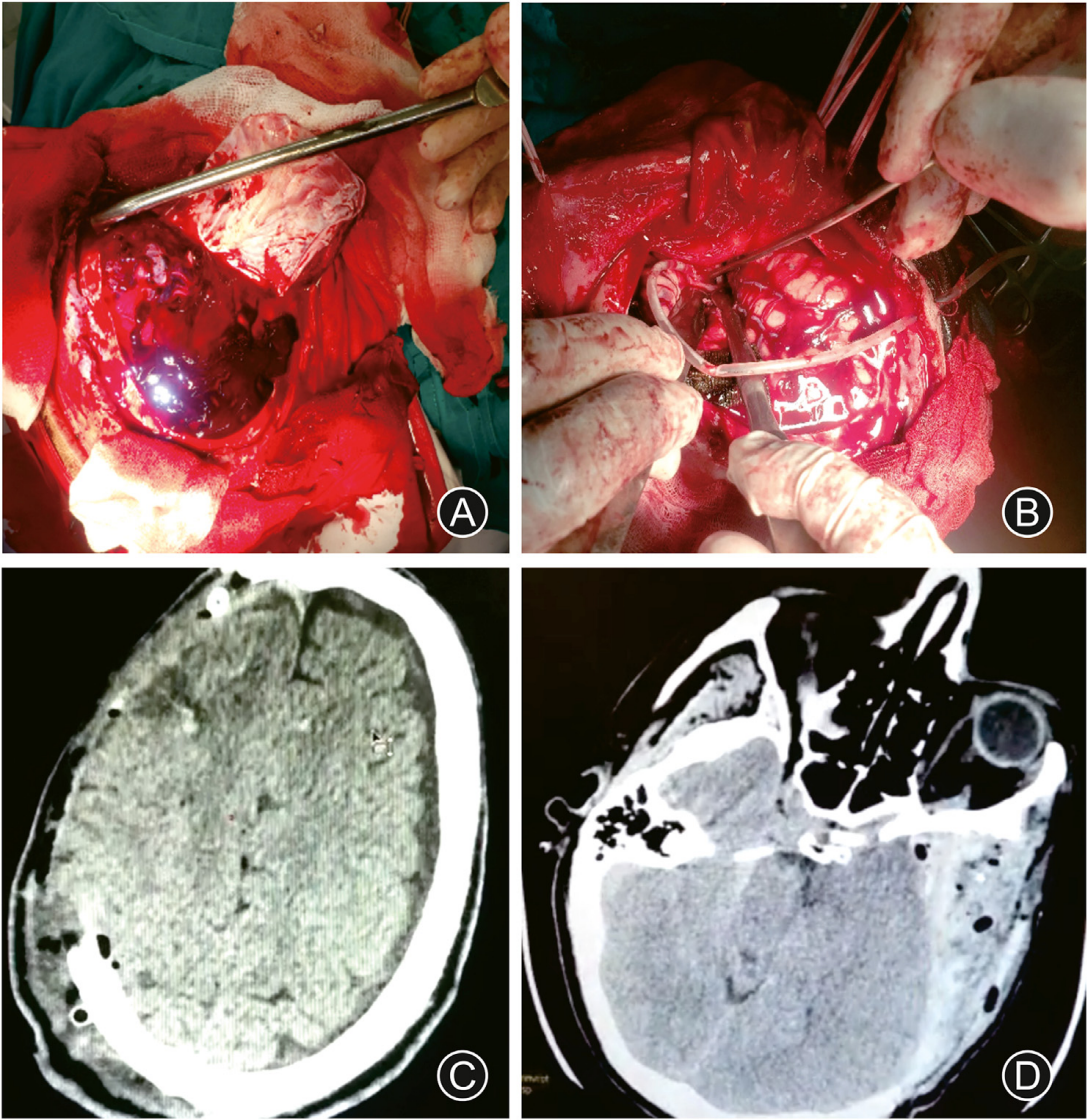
Intraoperative images and outcome. (A) After dural incision, the subdural blood clot covering the swollen and tight brain could be appreciated. (B) A temporary external drain was placed into the basal cistern, causing immediate brain relaxation. Postoperative axial CT showing the decompressive flap (C) and the drainage tube draining CSF from the basal cistern (D).
